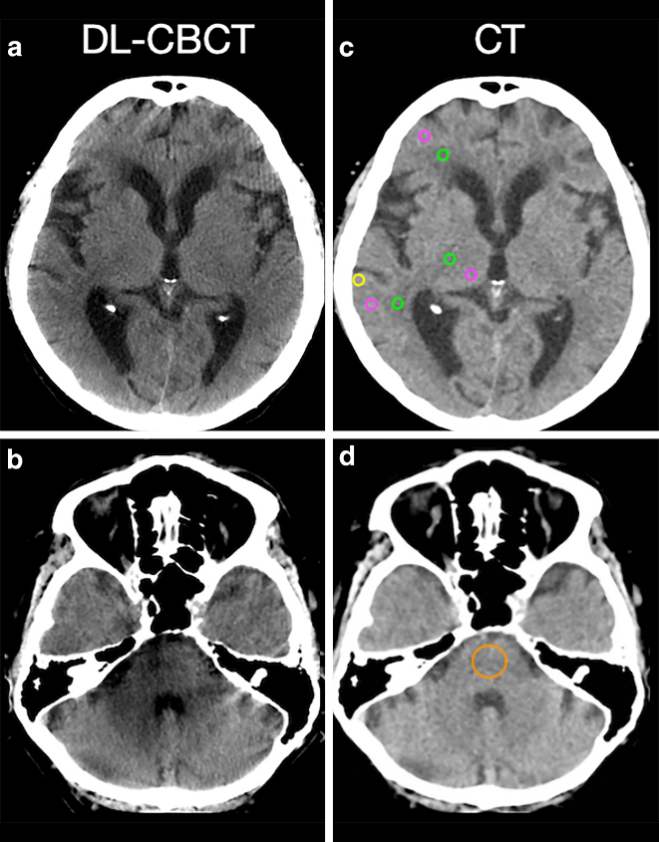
Axial non-contrast images of a participant with ischemic stroke. c, d show CT images with ROIs in the asymptomatic hemisphere and in the posterior fossa: gray matter (*Magenta*), adjacent white matter (*Green*), adjacent to the calvarium (“Subcalvarial”, *Yellow*) and in the posterior fossa (*Orange*). ROIs were automatically matched to the identical position in the DL-CBCT 75 keV images (a, b). All W/L: 75/25, 5 mm slices with average intensity projection. *DL-CBCT* Dual-layer cone-beam CT, *ROI* Region of interest
